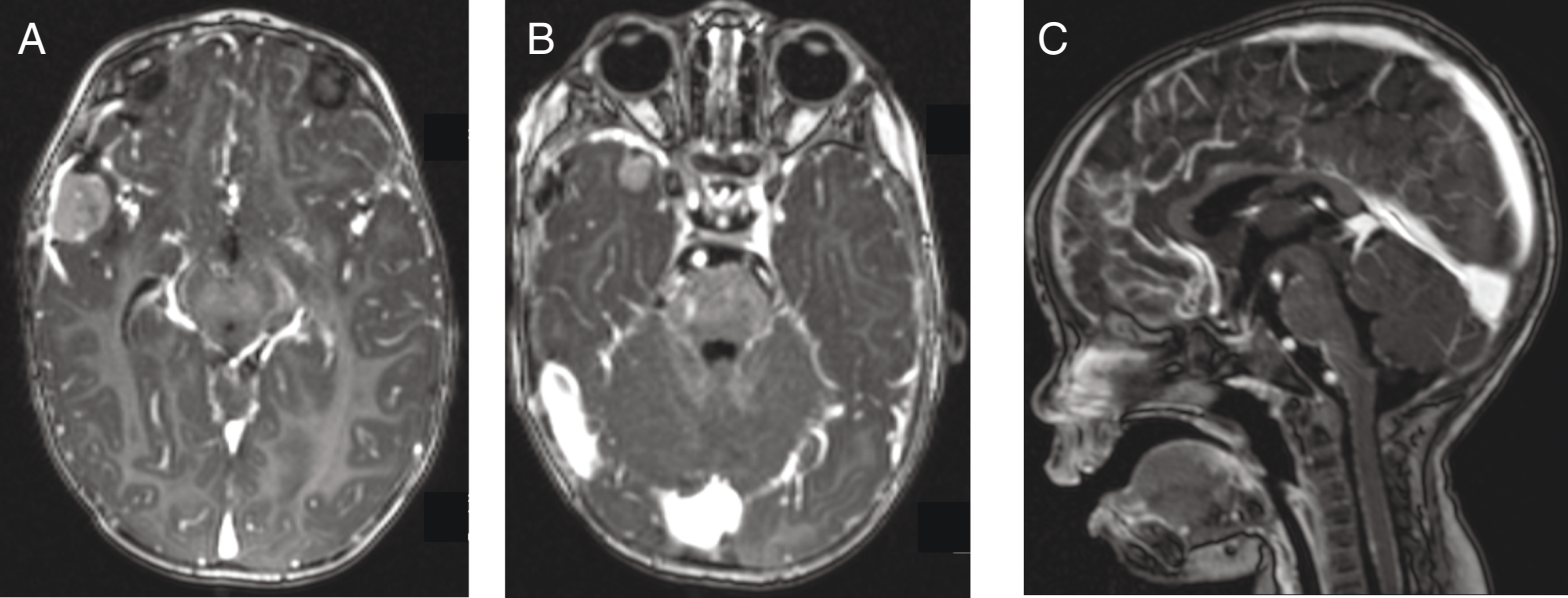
(A) T1-weighted axial post-contrast MRI at the time of first relapse demonstrating 2.2 × 1.8 cm right parietal lobe mass. (B) T1-weighted axial post-contrast MRI demonstrating 1.1 × 1.1 cm right temporal polar area mass. (C) T1-weighted sagittal post-contrast MRI demonstrating leptomeningeal thickening and enhancement most notable along the midbrain and brainstem.
